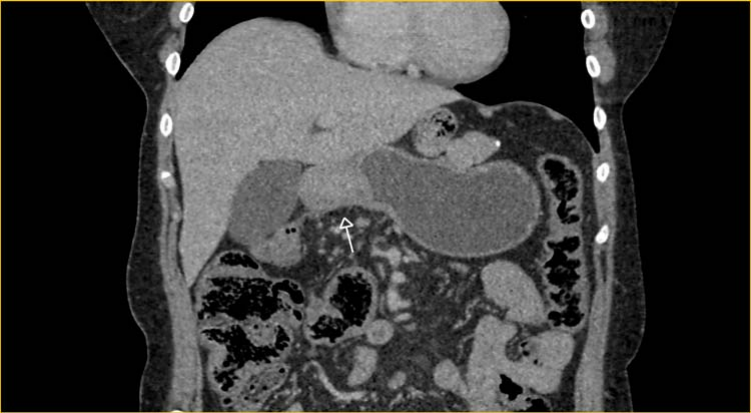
Portal phase coronal section image on CT showing excluded stomach distention with parietal thickening of the pylorus and antrum (arrow).
